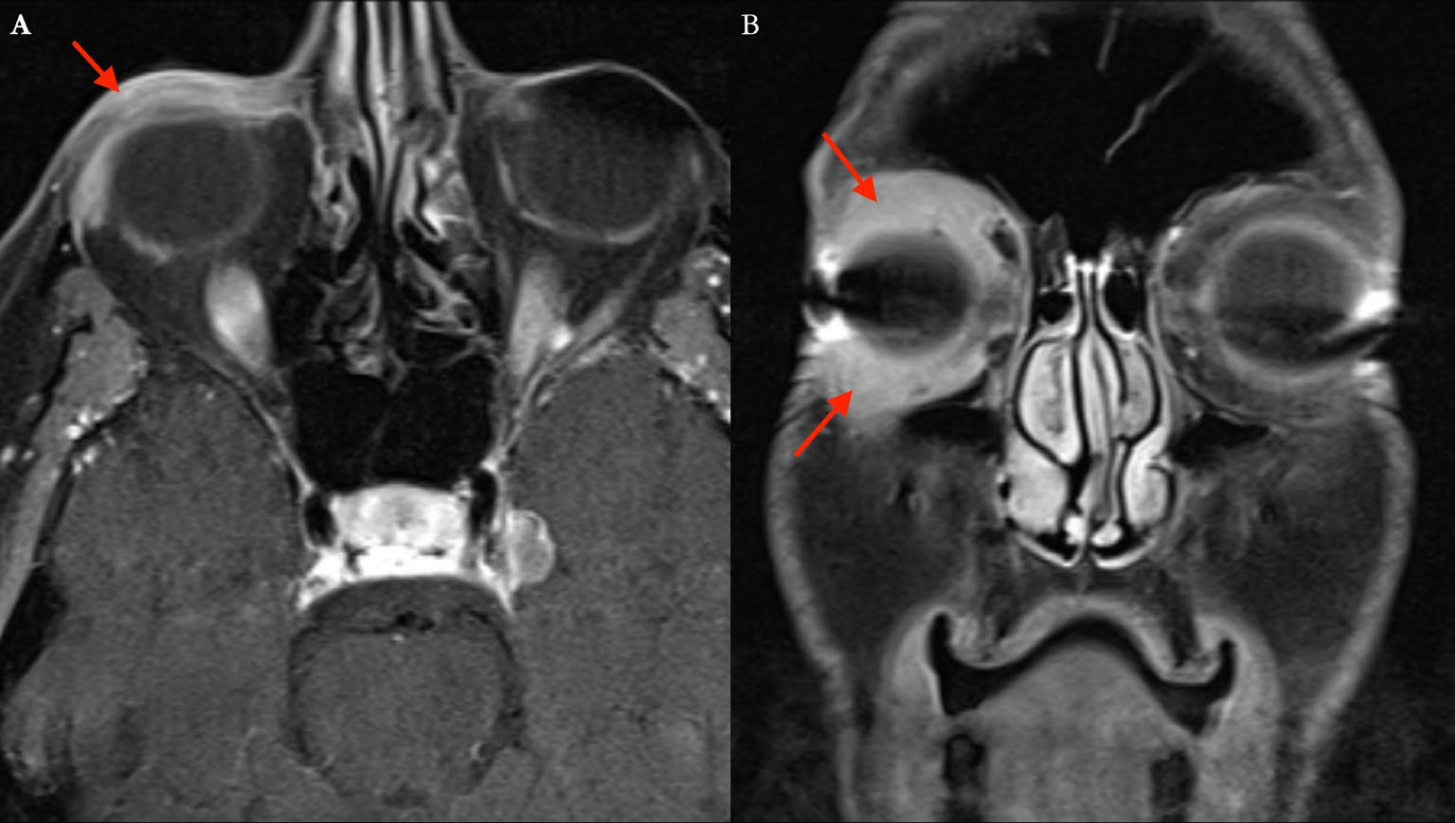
T1‐weighted, fat‐suppressed magnetic resonance images, axial (A) and coronal (B), demonstrate post‐contrast enhancing soft tissue thickening, involving the right upper and lower eyelids denoted by the red arrows.
